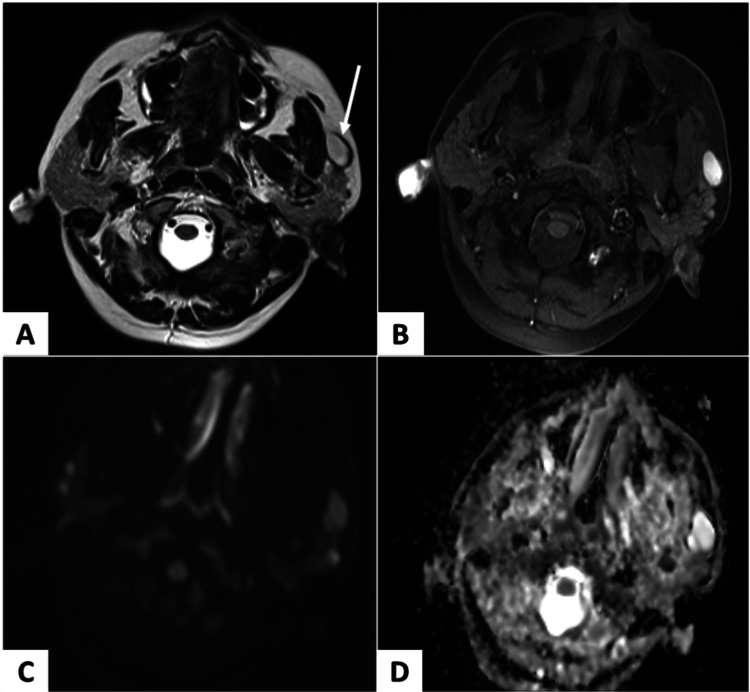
Evaluation using MRI. (A) T2-weighted axial image: A well-defined hyperintense lesion anterior to the left parotid gland (white arrow). (B) T1-weighted fat-suppressed image: Lesion appears hyperintense, most likely due to proteinaceous content within. (C) Diffusion-weighted imaging (DWI) & (D) apparent diffusion coefficient (ADC) images: A high signal was noted on the DWI image with no corresponding low signal on ADC images, suggestive of no restricted diffusion, hence unlikely to be epidermal inclusion cyst.
